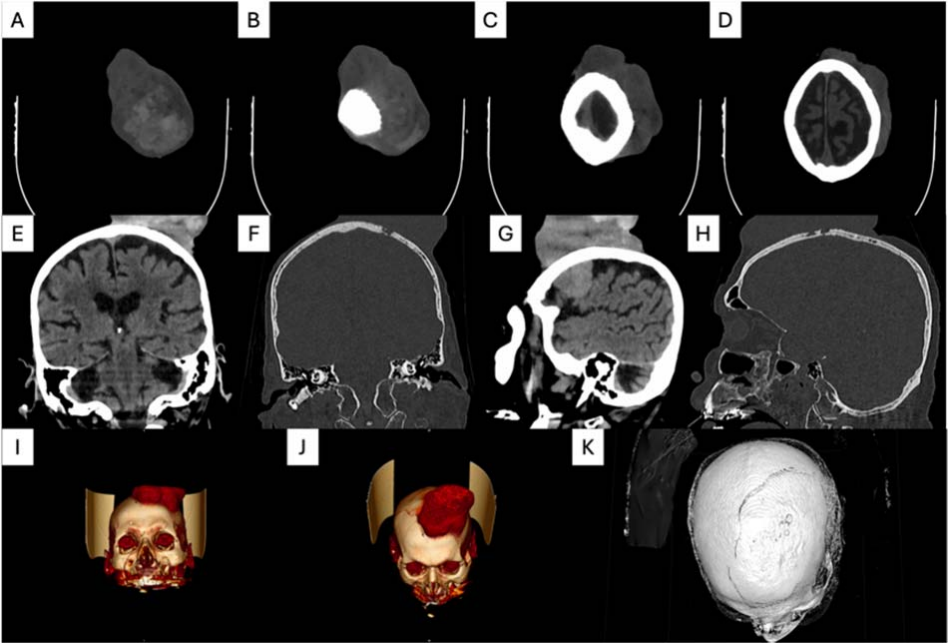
Computed tomography findings: An enhanced heterogeneous mass extending above the scalp exerting compressive effects on the brain and concomitant diffuse bone infiltration. (A–D) Axial view; (E and F) Coronal view; (G and H) Sagittal view; (I–K) 3-D reconstruction.
